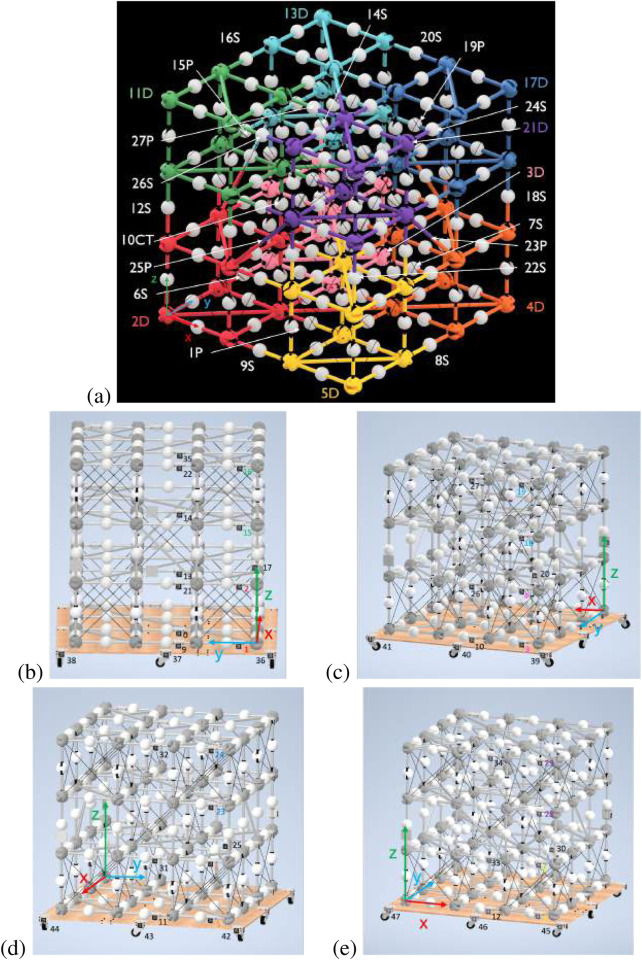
(a) BORG truss with labeled modules and axis at the outermost corner node on the first deployable truss, 2D (red). Qualifiers are used after the assembly number order to indicate whether the module is a close-out square (P), deployable (D), close-out strut (S), or the center truss deployable (CT). In addition, the corner deployable modules are color-coded in rainbow order to aid in quick identification during hardware trials. (b) Side 1 of the BORG truss. (c) Side 2 of the BORG truss. (d) Side 3 of the BORG truss. (e) Side 4 of the BORG truss.
